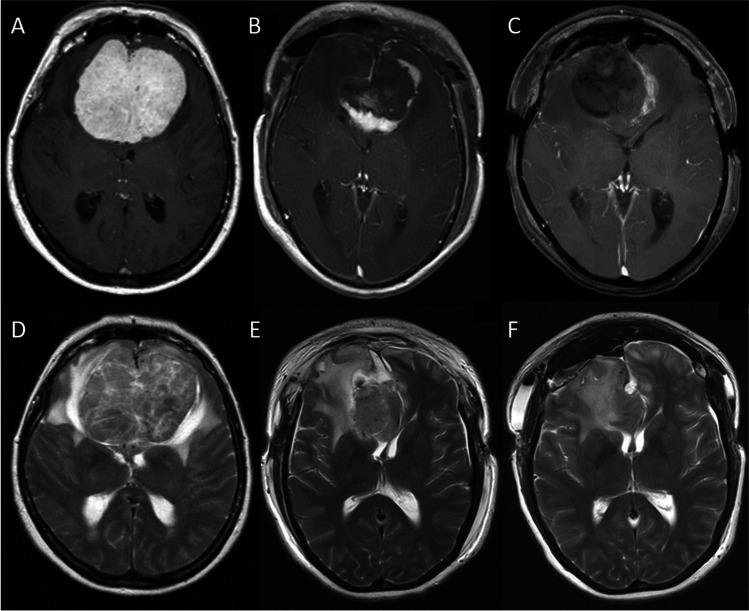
Axial MRI images. A, B, C, D, E, F: same sequences as in Fig. 1 each
